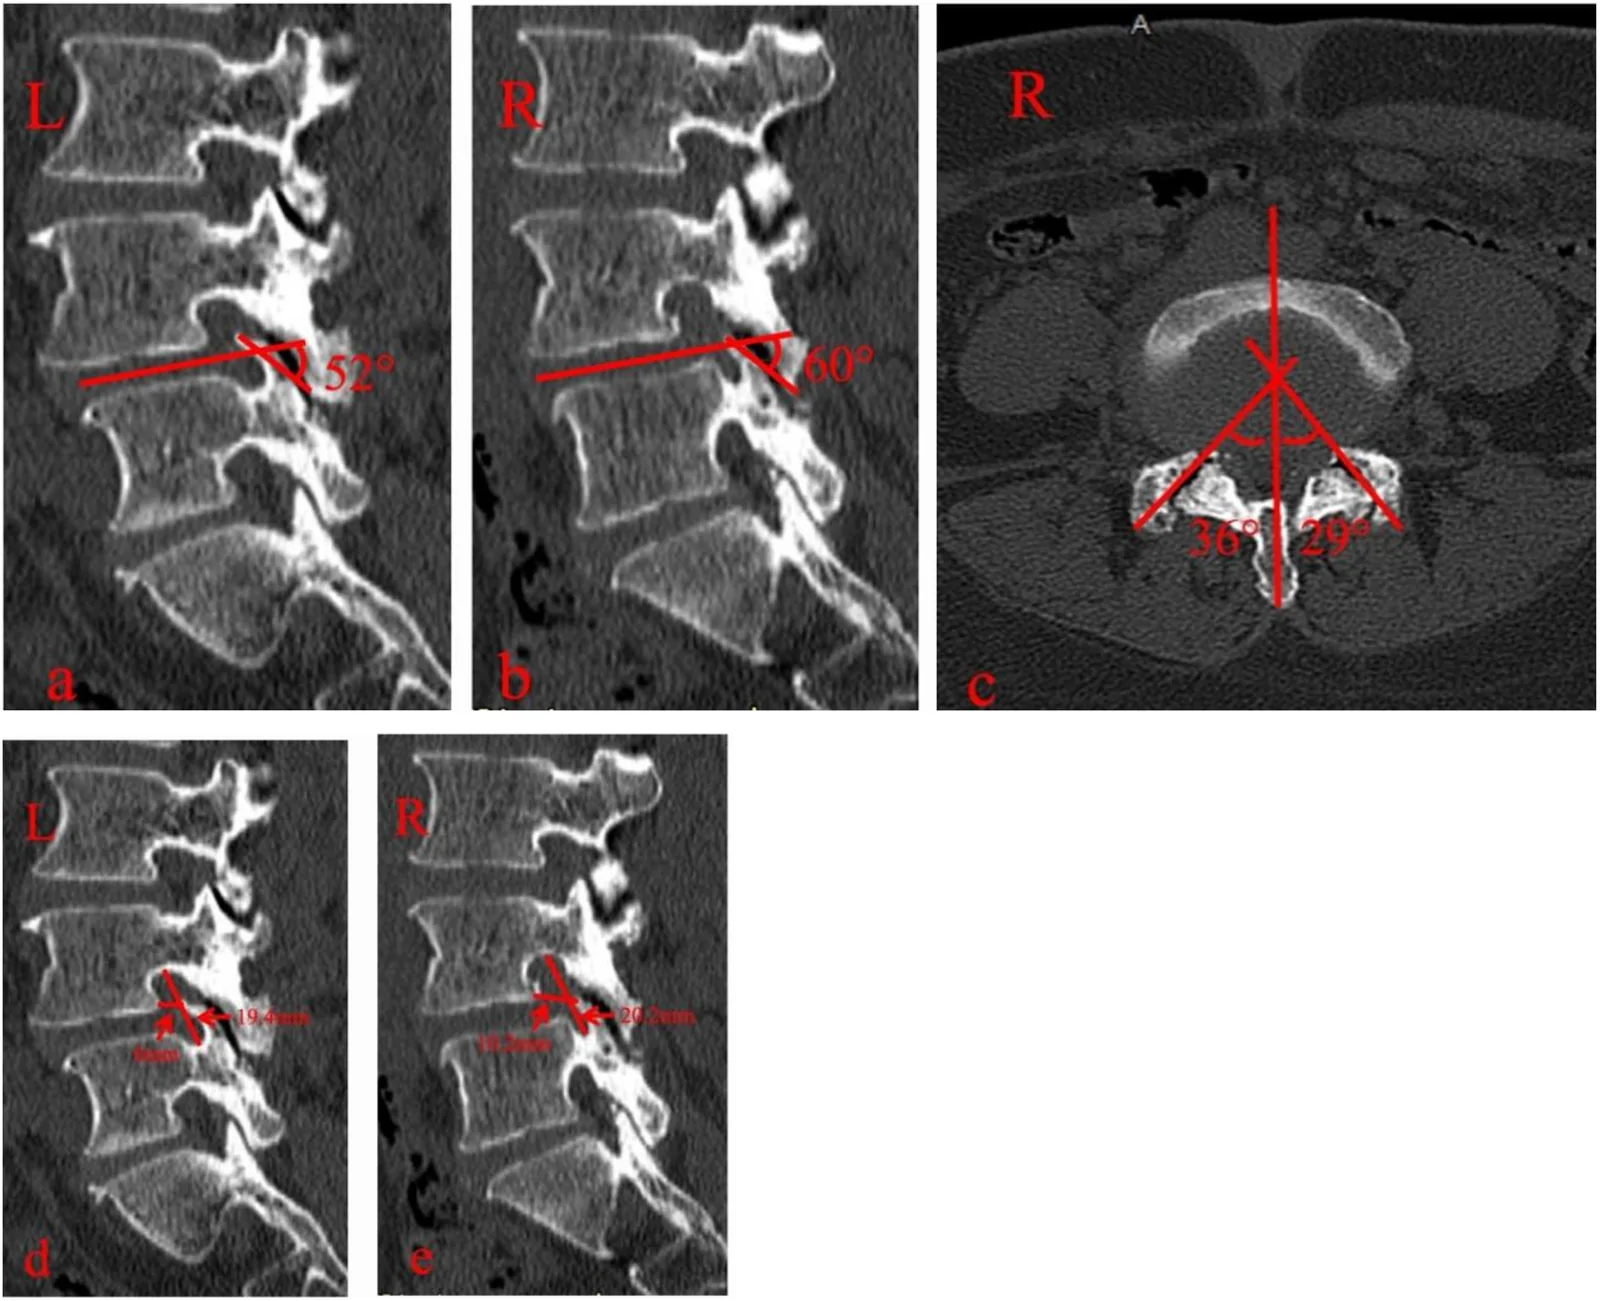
Preoperative CT scans demonstrated L4/5 facet joint degeneration and elongation of bilateral L5 superior articular processes (SAP). The difference of bilateral facet joint angles was 8° in the sagittal plane (a,b) and 7° in the axial plane (c) The width of the left neuroforamen was smaller than that of the right, while the heights were nearly equivalent (d,e).
